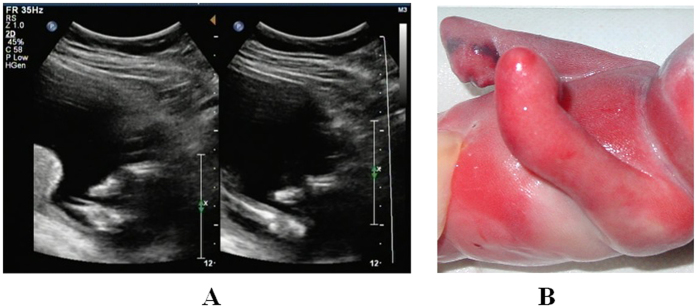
The absence of both radius was observed at a gestational age of 25 weeks. (A) Two-dimensional sonogram showing that both forearms are shortened, with only one long bone visible and with abnormal hand positioning (hook-like). The metacarpal bone plane could not be identified. (B) Macroscopic view of the fetus showing the shortening and bending of both arms. Additionally, both hands were in radius flexion, and abnormalities in the palm and fingers were observed.
